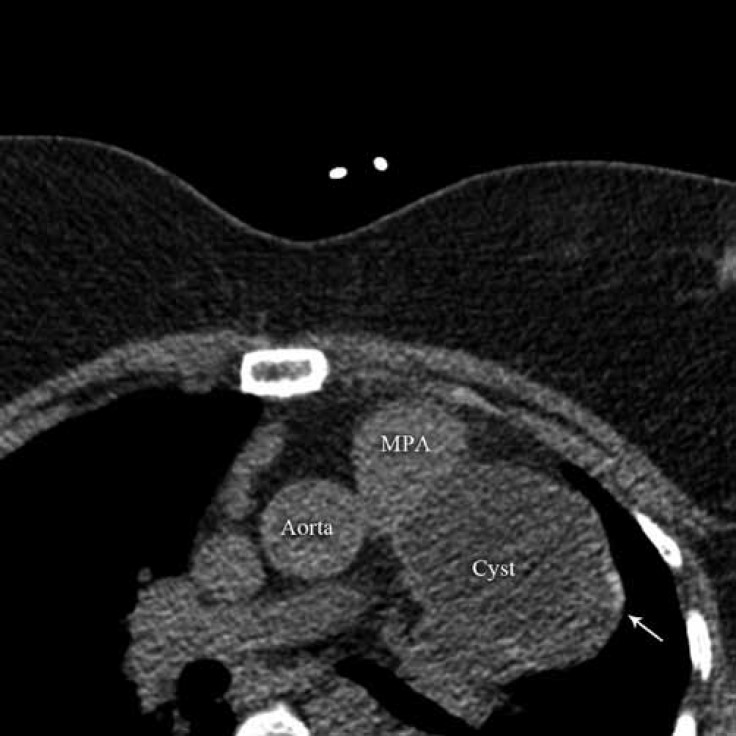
Cross-section of the mediastinum in the computed tomography angiography reveals a large cyst (arrow).
